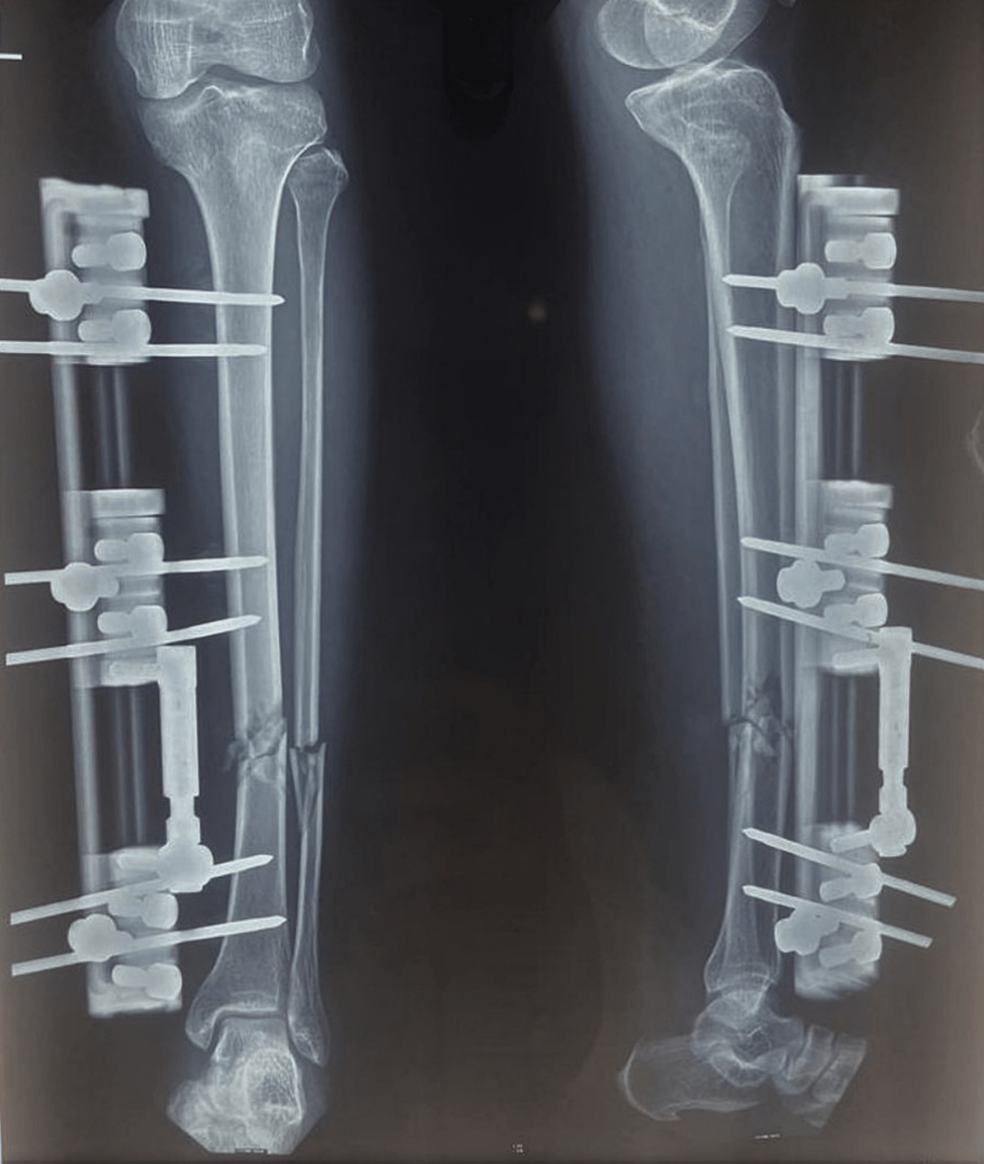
Immediate postoperative X-ray (anterioposterior and lateral view)
